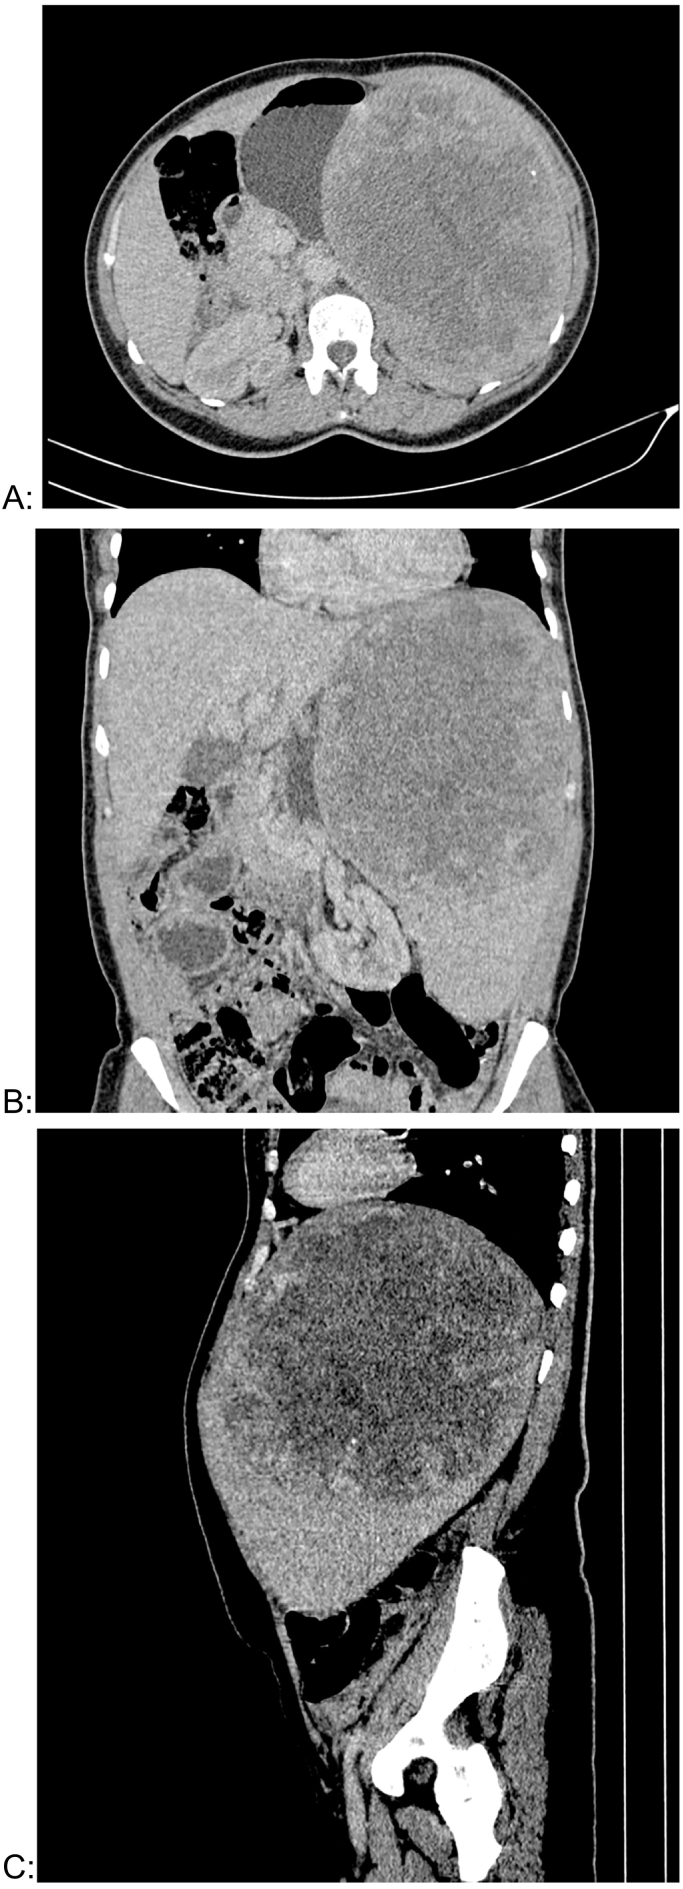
A Computed tomography imaging showing the spleen mass.
